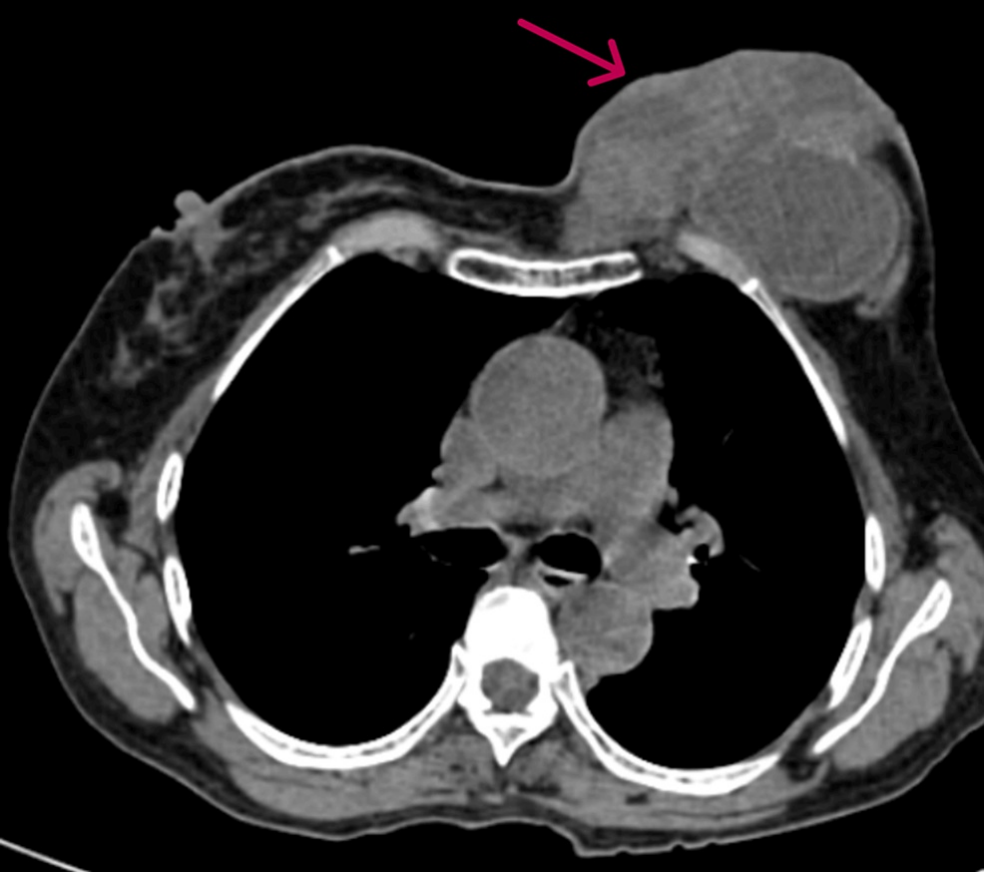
Contrast-enhanced CT of the thorax of the patient The image shows a heterogeneously enhancing, multilobulated, ulceroproliferative lesion in the left breast parenchyma with adjacent fat stranding and multiple non-enhancing areas of central necrosis CT: computed tomography
